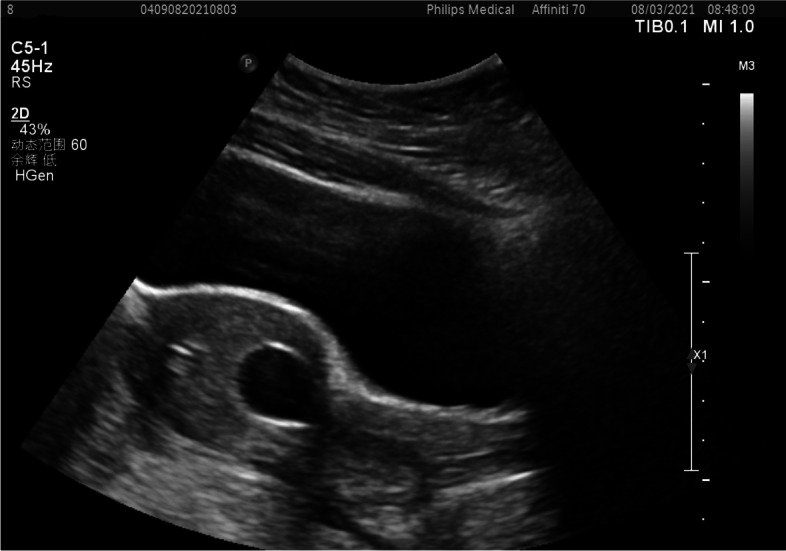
Ultrasonographic examination showed that Foley catheter had been placed correctly with the balloon inflated in the lower segment of the uterine cavity and myometrium of the uterine isthmus was continuous
